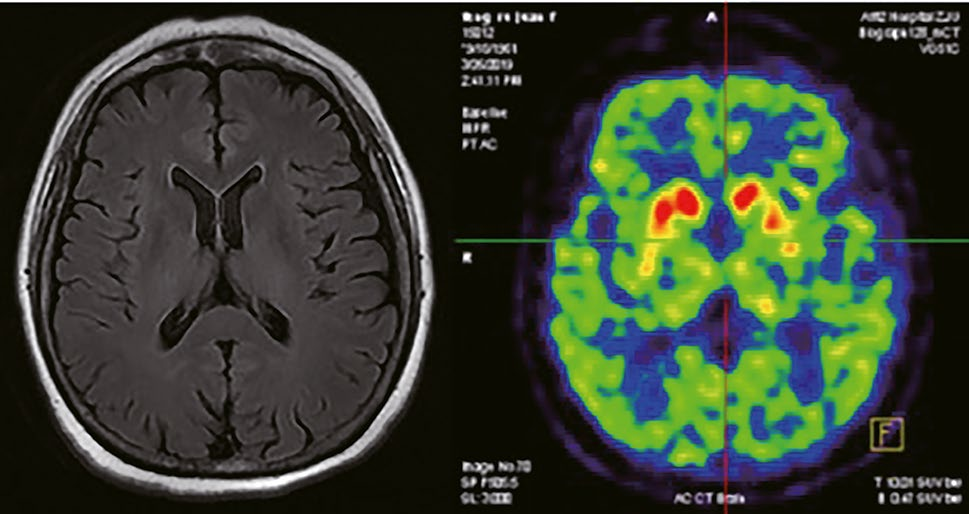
No dialted ventricles or white matter lesions found on MR axial T2‐weighted‐Fluid‐Attenuated Inversion Recovery (T2‐FLAIR). DAT revealed bilateral striatum dopaminergic deficit, more prominent reduction observed in left body of caudate
